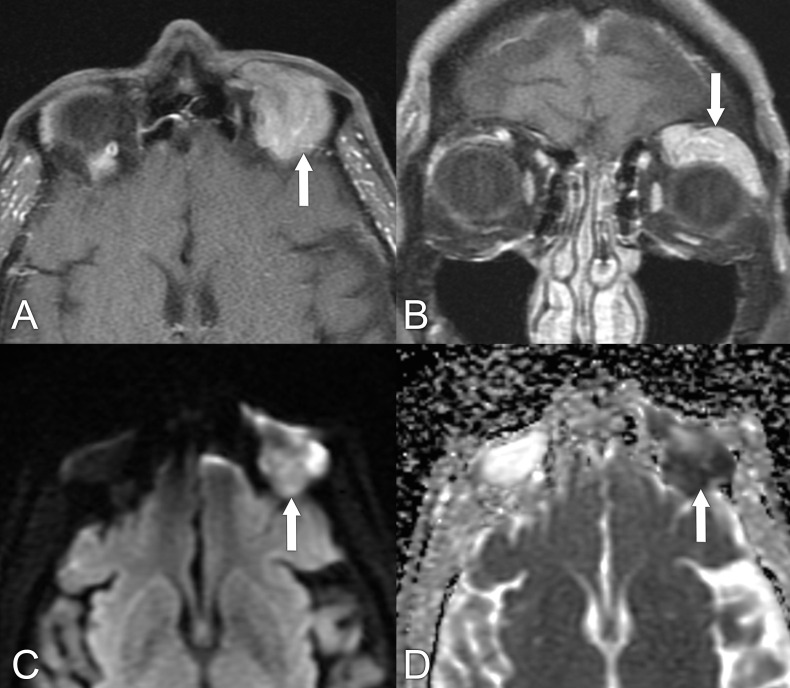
A 76-year-old man with left conjunctival mucosa-associated lymphoid tissue (MALT) lymphoma presented with ptosis. (A, B) Axial and coronal T1-weighted postcontrast MRIs with fat suppression show a homogeneously enhancing mass in the superior left orbit that molds around the globe (arrows). (C, D) Axial diffusion-weighted and apparent diffusion coefficient MRIs show restricted diffusion within the mass, with signal hyperintensity and hypointensity, respectively (arrows).
